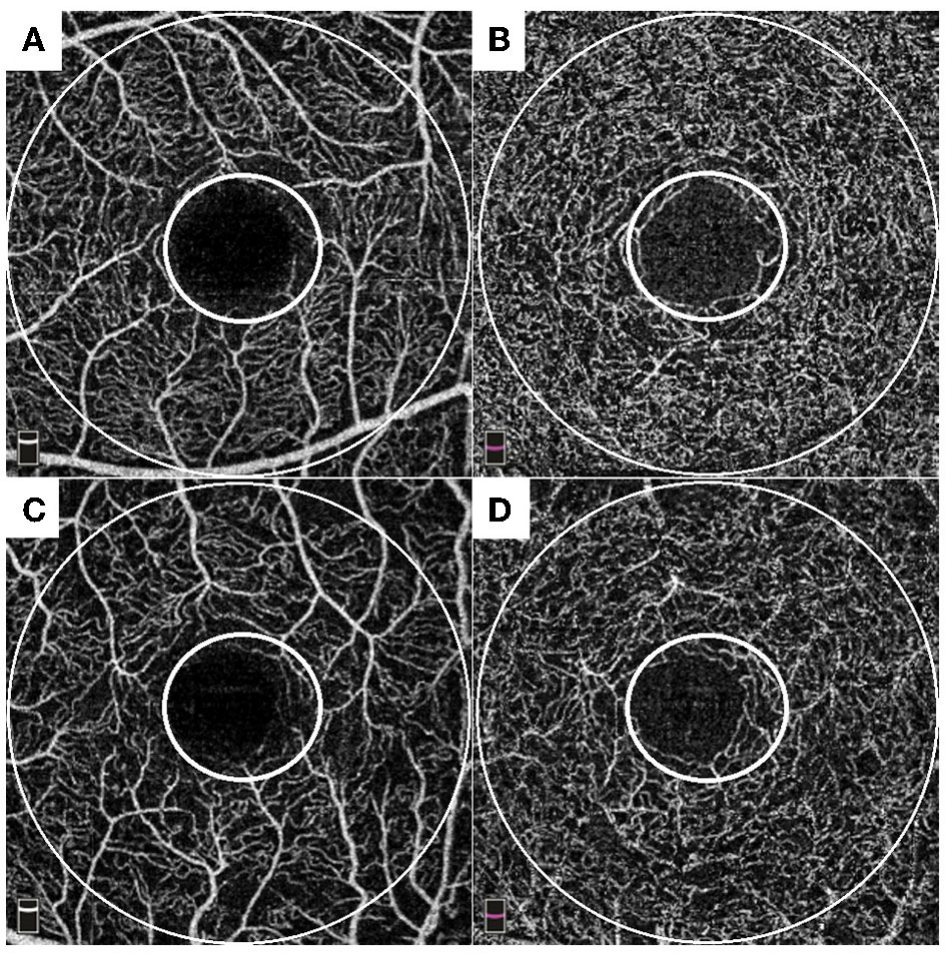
Representative cases of an age-sex matched control (A,B) and a patient with subclinical atherosclerosis (C,D). Each of small and large circles denote foveal and parafoveal area. Control: A 56-year- old male patient with 18-years history of diabetes showed 0.63 mm of carotid intima media thickness (IMT) with plaque-free in carotid ultrasonography (US). He had well-preserved foveal VD around foveal avascular zone FAZ (49.7%), parafoveal VD in superficial capillary plexus (49.3%), and parafoveal VD in deep capillary plexus (50.3%) in OCTA. A patient with subclinical atherosclerosis: a 57-year- old male patient with 5-years history of diabetes showed 1.11 mm of carotid IMT with plaque in carotid US. He had impaired foveal VD around FAZ (45.9%), parafoveal VD in superficial capillary plexus (40.8%), and parafoveal VD in deep capillary plexus (46.5%) in OCTA.
